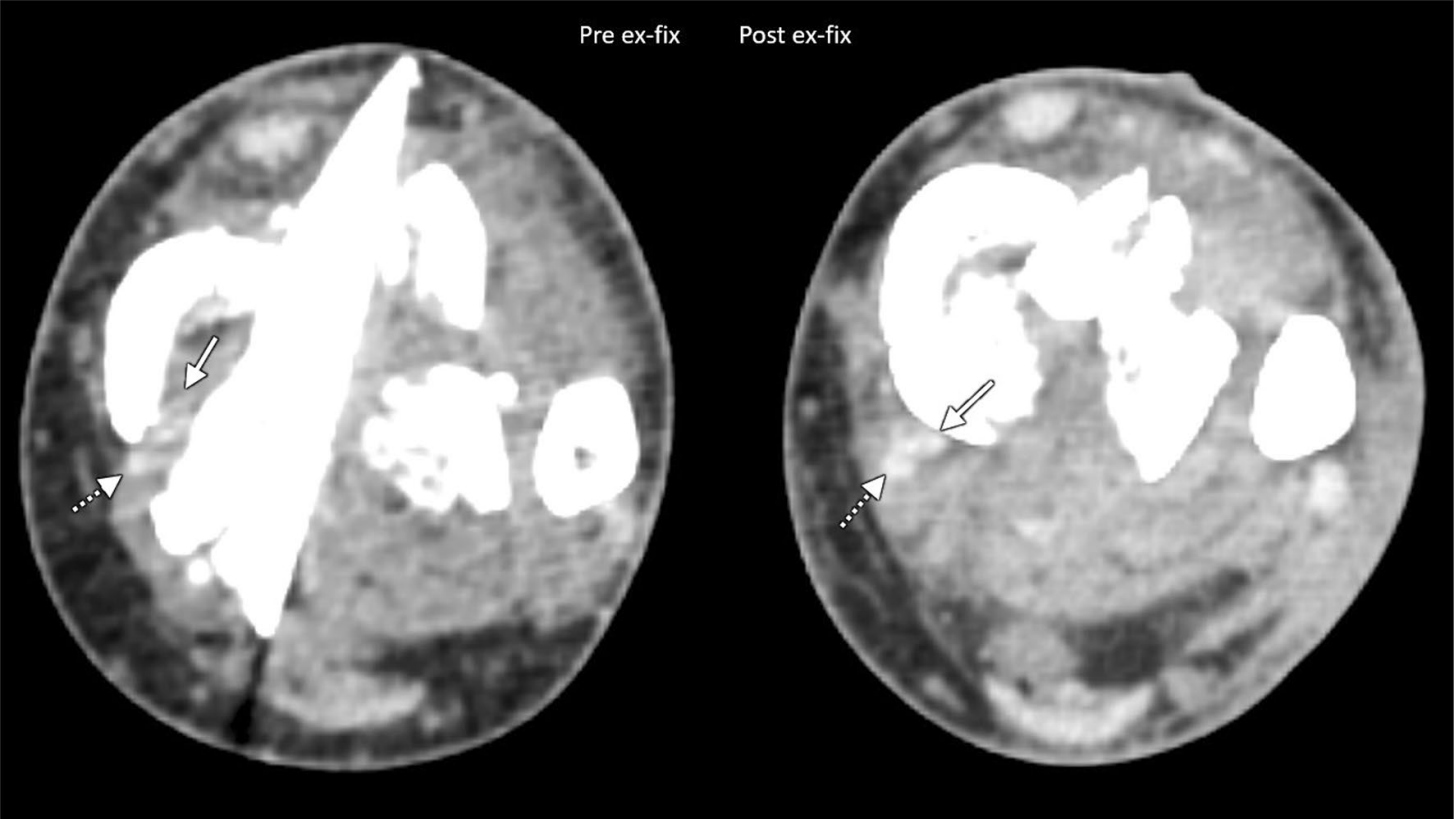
Thirty-one-year-old man who fell from the US border fence, with AO/OTA 43C3.3 pilon fracture (Ruedi/Allgower type 3). Initial axial trauma CT angiogram (left) shows entrapment of the posterior tibial tendon (solid arrow) and flexor digitorum longus tendon (dotted arrow) within the fracture. Post-SEF CT (right) shows release of both tendons
